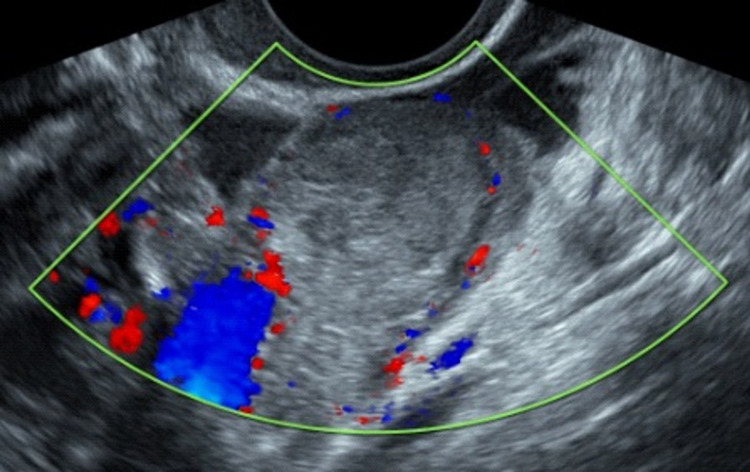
Transvaginal ultrasound scan, showing the inhomogenous mass with classical findings of a tubal ectopic pregnancy with increased surrounding vascularity.
